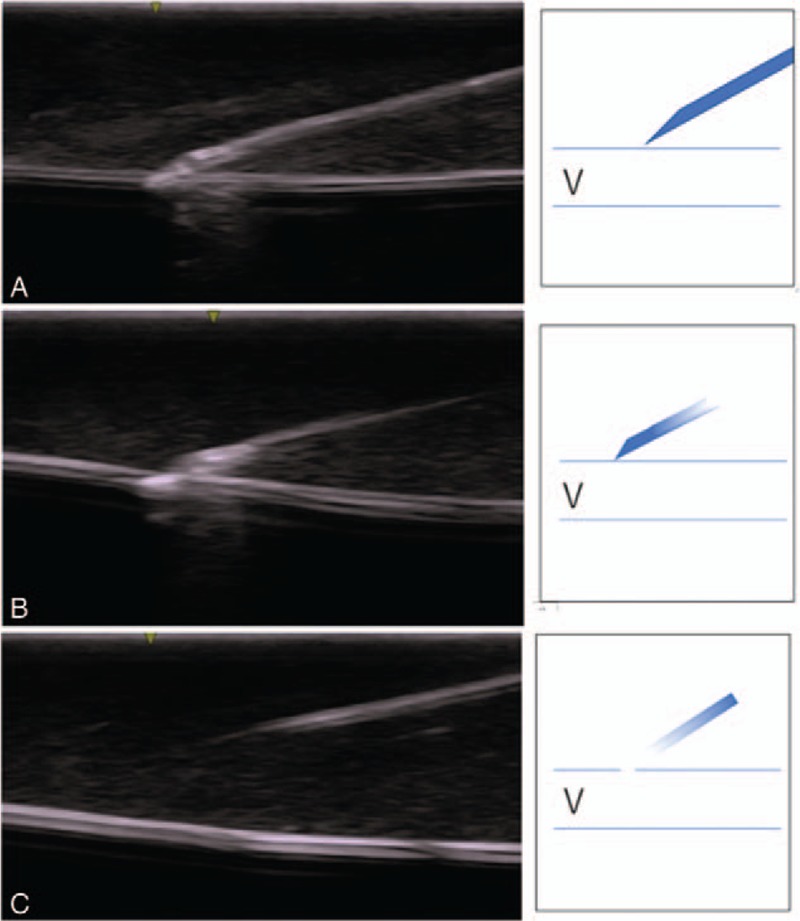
Needle tip visualization. Ultrasound experts observed the recorded ultrasound views and evaluated whether the needle tip could be classified as “identified” (clearly visible, or visible) or not (“unidentified”). Typical images judged as clearly visible, visible, and unidentified are shown above. Panel A: Clearly visible: Needle tip and shaft can be seen in full length. Panel B: Visible: Needle tip and shaft can be seen partially. Panel C: Unidentified: Needle tip cannot be observed. The shaft can be seen partially.
